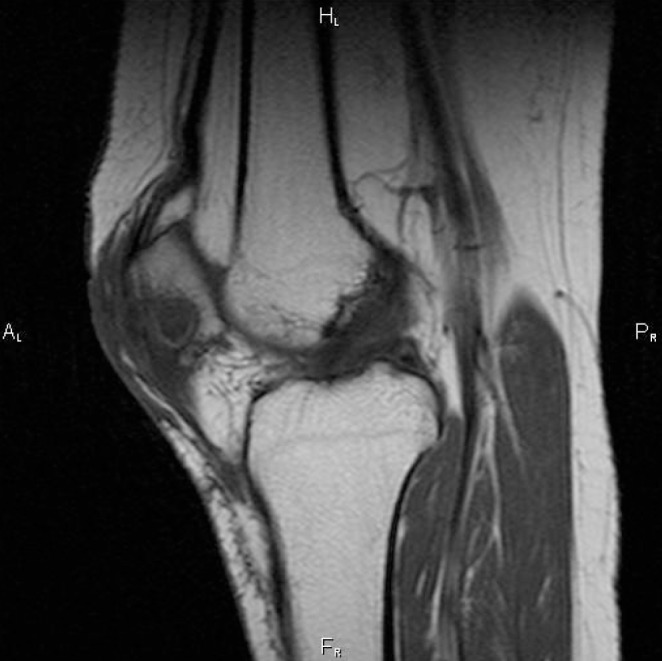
T1 weighted magnetic resonance imaging showing a 16mm × 12mm well defined lucency with central calcific densities, suggesting chronic osteomyelitis with sequestrum
